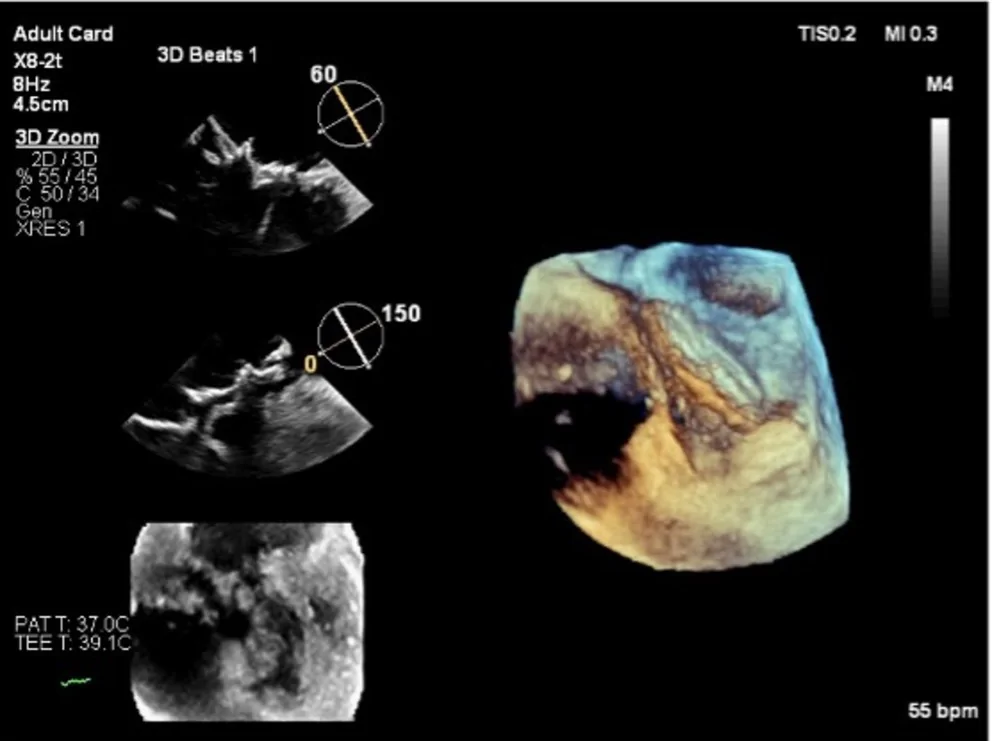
Three‐dimensional transoesophageal echocardiography showing the right disk of the device.
